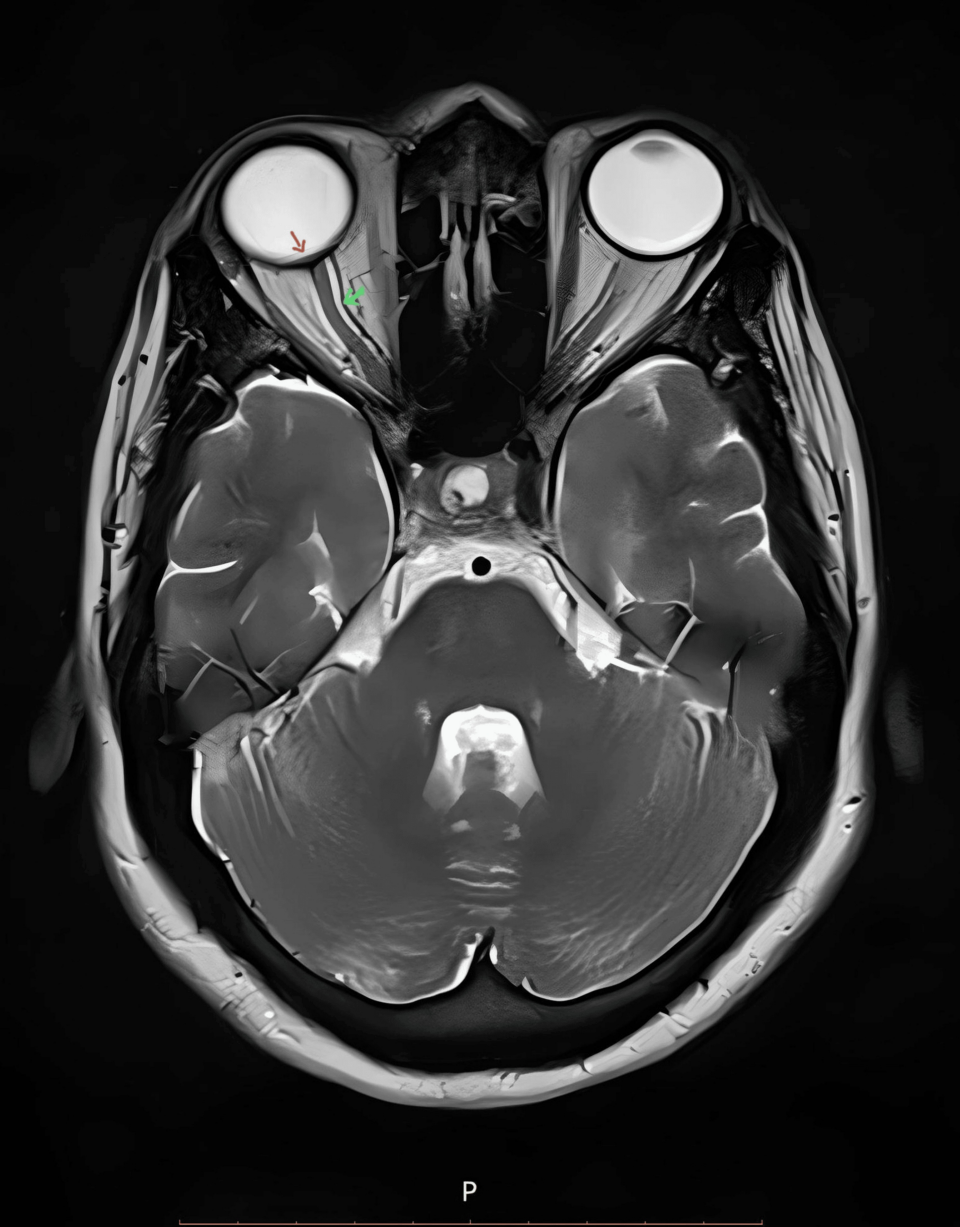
Axial T2-weighted MRI of the brain at the level of the optic nerve, displaying hyperintense CSF around the optic nerve (green arrow) and bulging of the optic nerve papillae (red arrow), indicative of papilledema
